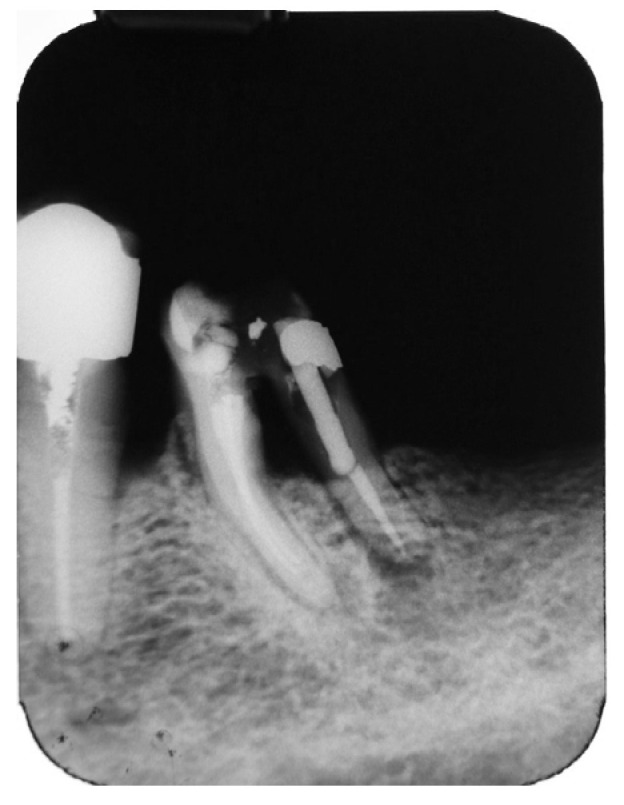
Postsurgical X-ray after the built-up of the tooth and removal of the distal-buccal root of tooth #36.
